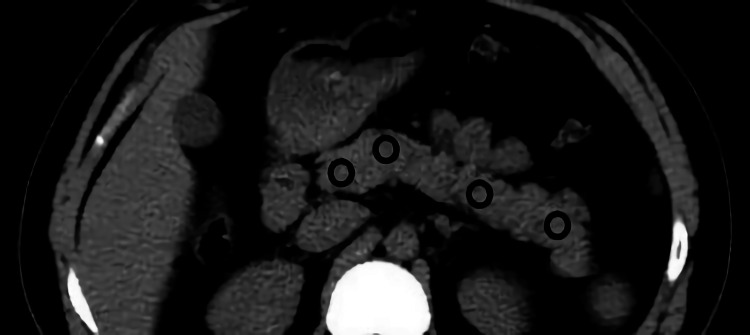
Measurement Measurement of the pancreatic head, neck, corpus, and tail densities by placing a circular region of interest on non-contrast axial CT images CT: computed tomography
